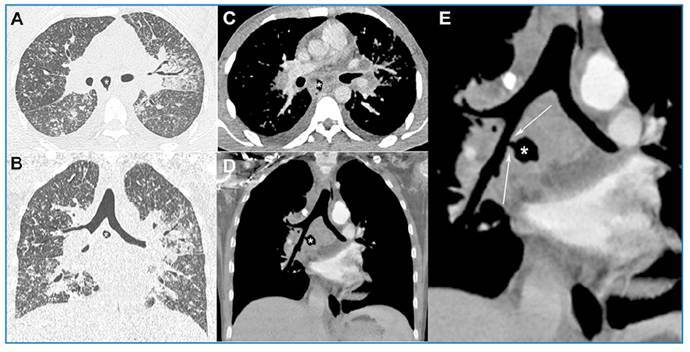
Axial (A) and coronal (B) chest computed tomography (CT) scans with pulmonary windows show micronodular infiltrate, paracardiac consolidations, and an air cavity of subcarinal localization (asterisk). Axial (C) and coronal (D) CT scans with mediastinal windows and detail (E) show enlarged subcarinal lymphadenopathy with necrosis and the fistulous trajectory of the air cavity (necrotic lymph node), draining to the bronchus intermedius (arrows in E).
